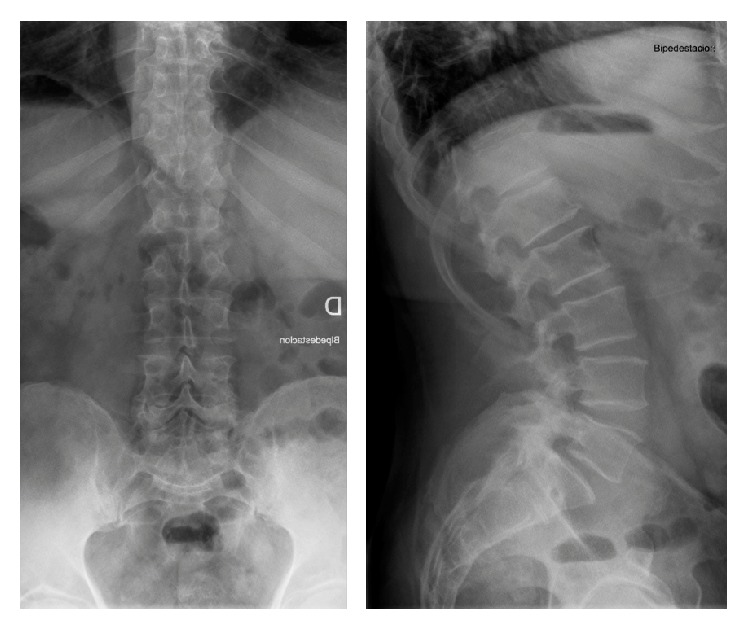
Preoperative anteroposterior and sagittal X-ray. Spondylolisthesis L5-S1 grade I.
